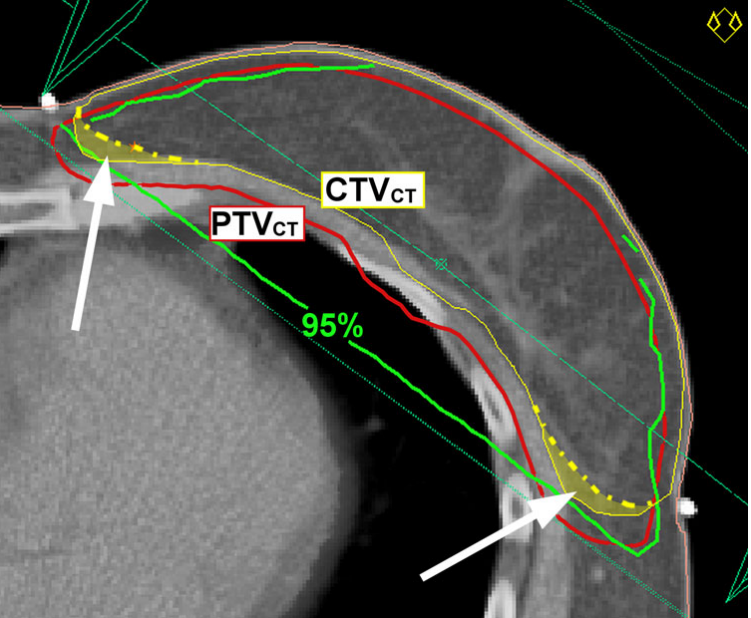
Dose-distribution conventional breast beams relative to CT-based breast target volumes. Representation of 95%-isodose (green) resulting from conventional breast beams and computed tomography (CT)-based clinical target volume (CTV) and planning target volume (PTV). Note the areas of PTV (red) not covered by 95%-isodose when conventional beams are used. Under-dosage of PTV is caused by including additional tissue (marked yellow-wash areas) into the CTV.
